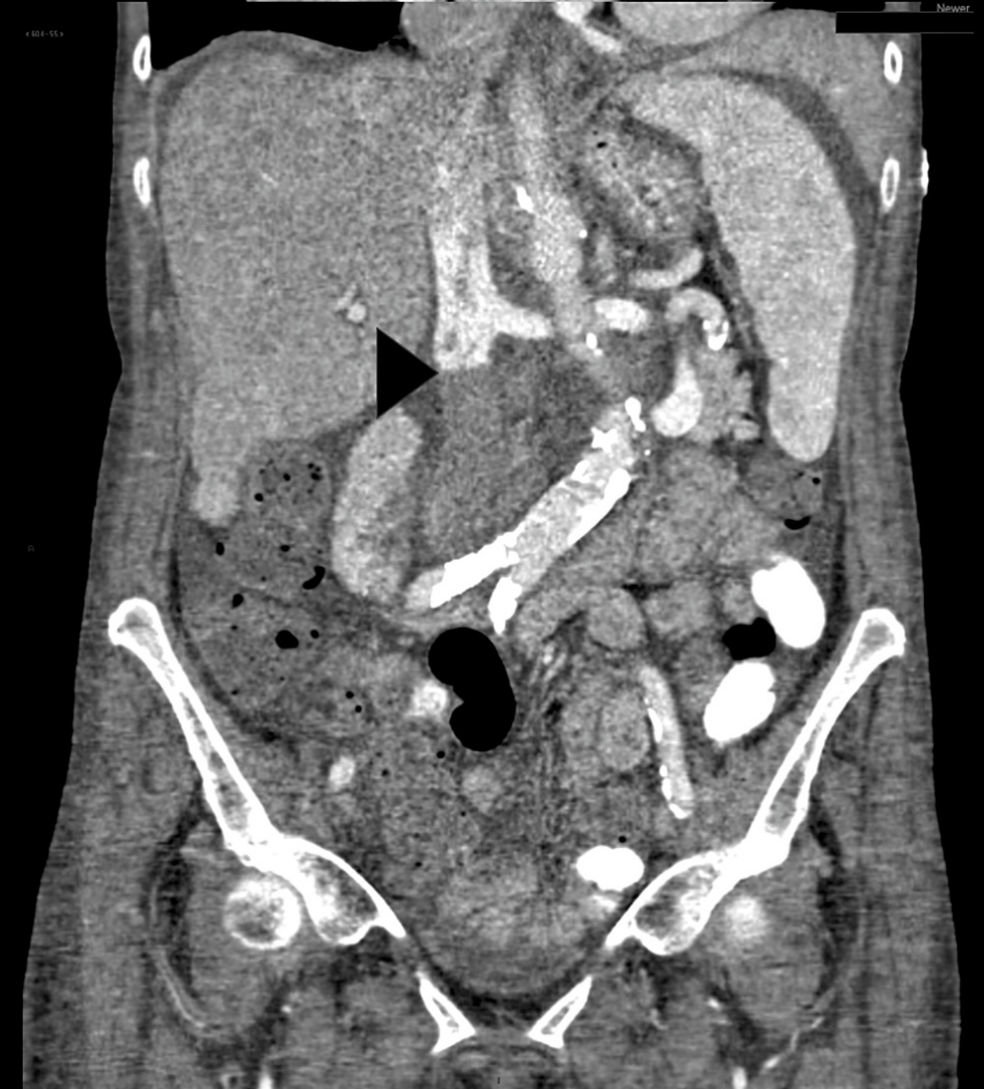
CT abdomen with contrast in the coronal plane showing a large thrombus completely occluding the inferior vena cava (black arrowhead) as well as mass/thrombus extension proximally.
